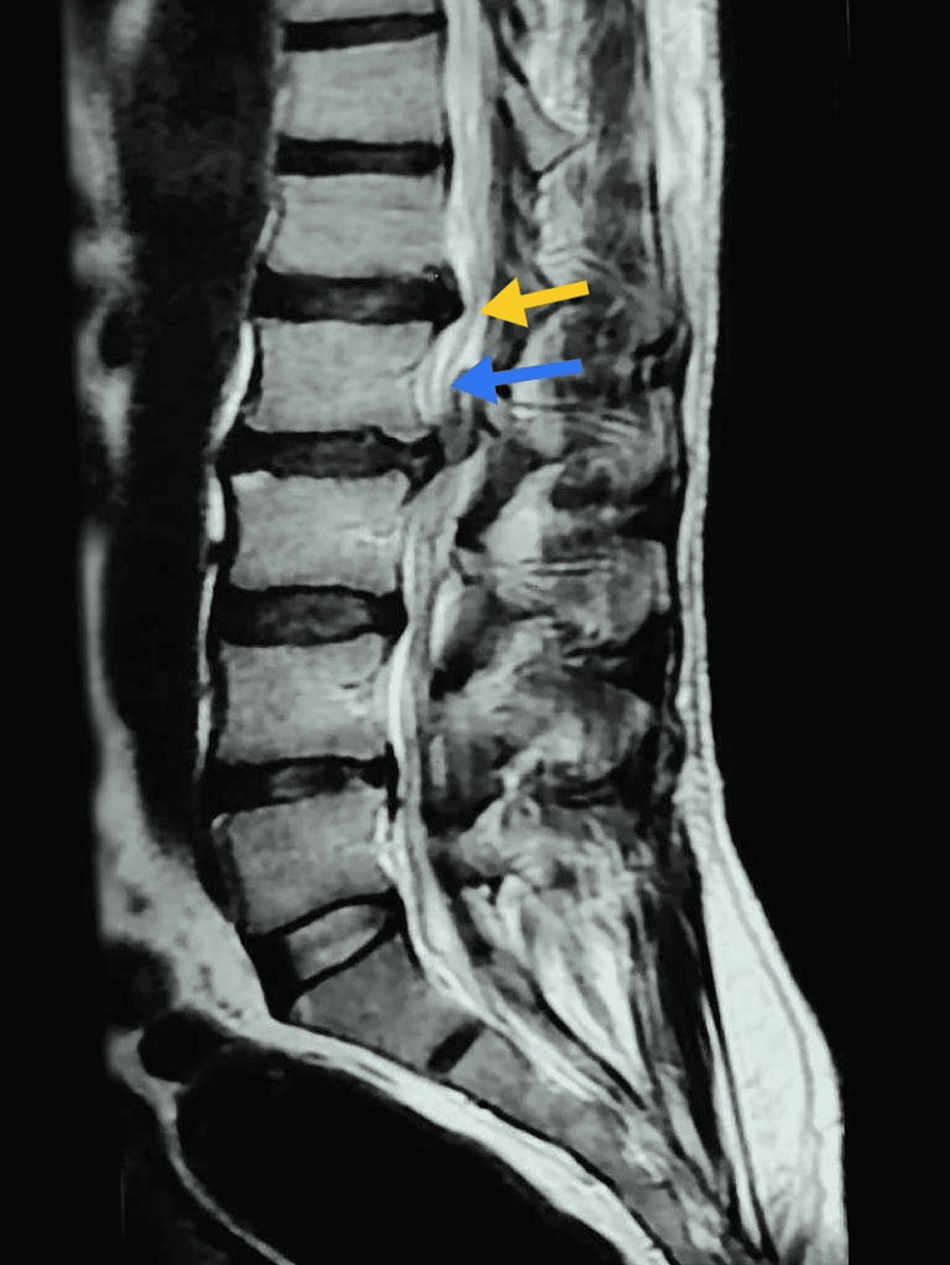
T2-weighted image of a sagittal view of the lumbar spine showing posteriorly migrated disc (shown by an yellow arrow) compressing the nerve root and thecal sac (shown by an blue arrow)
